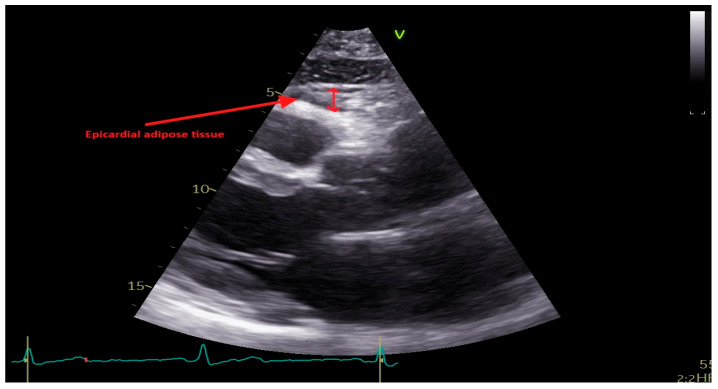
Measurement of epicardial fat thickness by transthoracic echocardiography.
